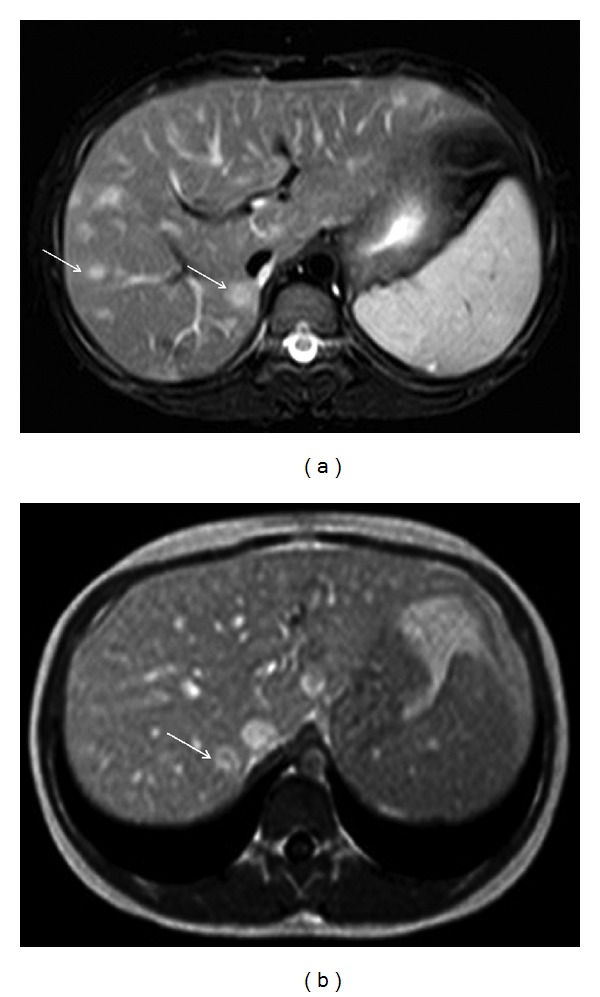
Follow-up abdominal MRI, axial images through the liver: the lesions (arrows) seen in Figure 1 present signs of maturity. Their margin is more distinct (STIR image in (a)), as edema has resolved, and the peripheral enhancing rim is better defined (T1W image after IV contrast administration in (b)).
